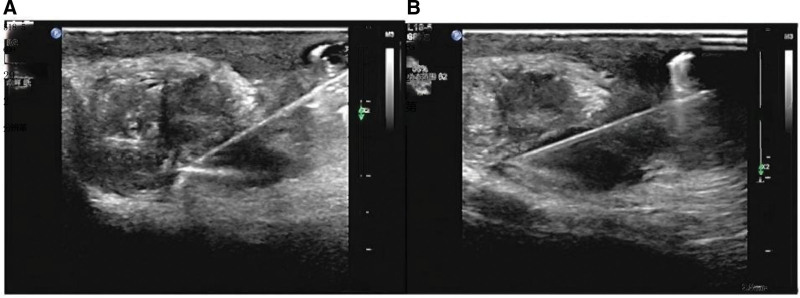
(A, B): The representative ultrasound imaging clearly reveals the hypoechoic nodules in the epididymis tail, local rupture and scrotum involvement. The ultrasound also clearly directed the puncture needle when performing the biopsy at the focus.
